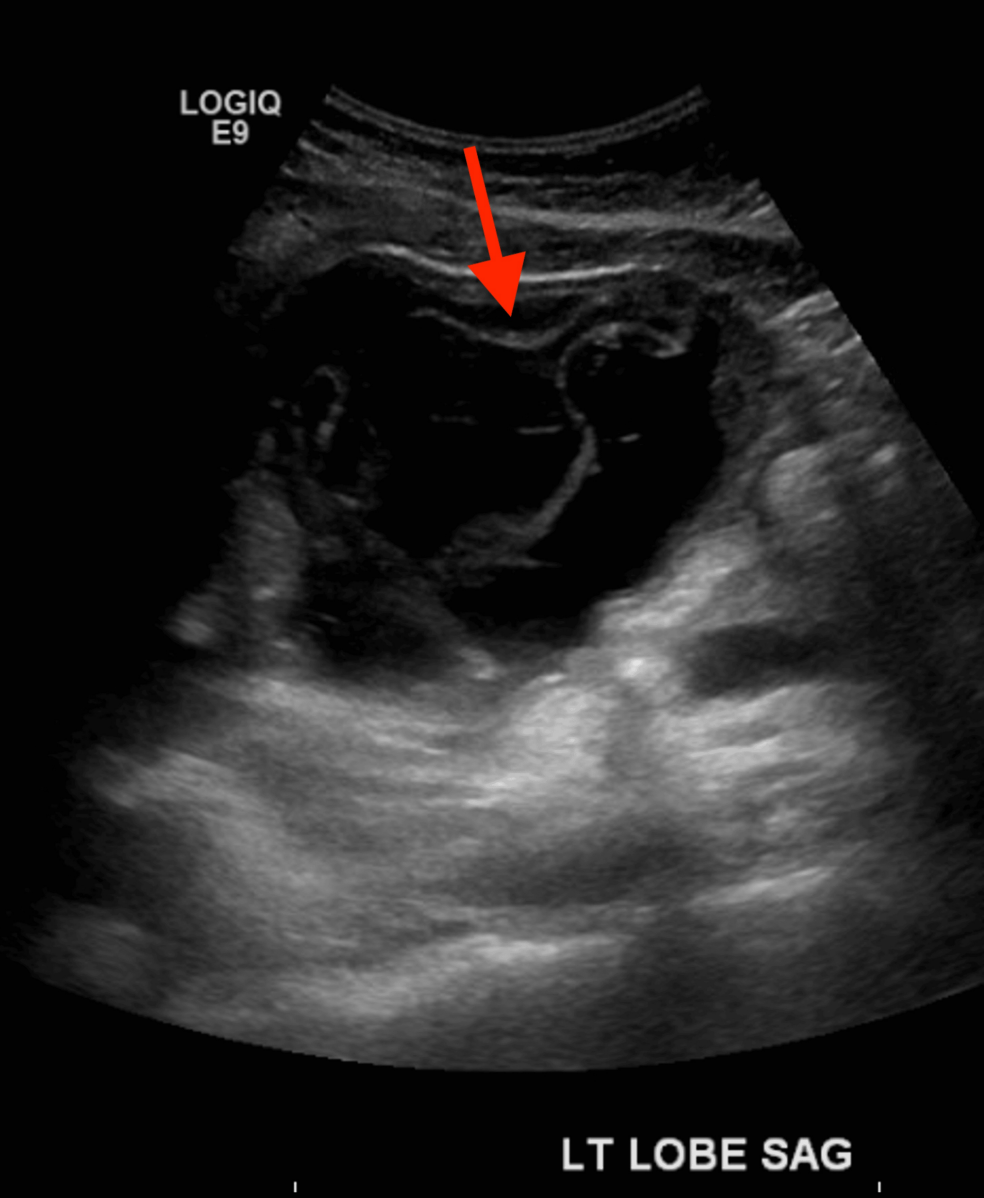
Abdominal ultrasound Abdominal ultrasound demonstrating a complex cystic structure in the left hepatic lobe measuring 11.6×8×8 cm (red arrow), consistent with a hydatid cyst. The size and morphology, along with the patient's clinical history, raised concern for potential rupture, guiding the decision for PAIR intervention. PAIR - puncture, aspiration, injection, and re-aspiration
